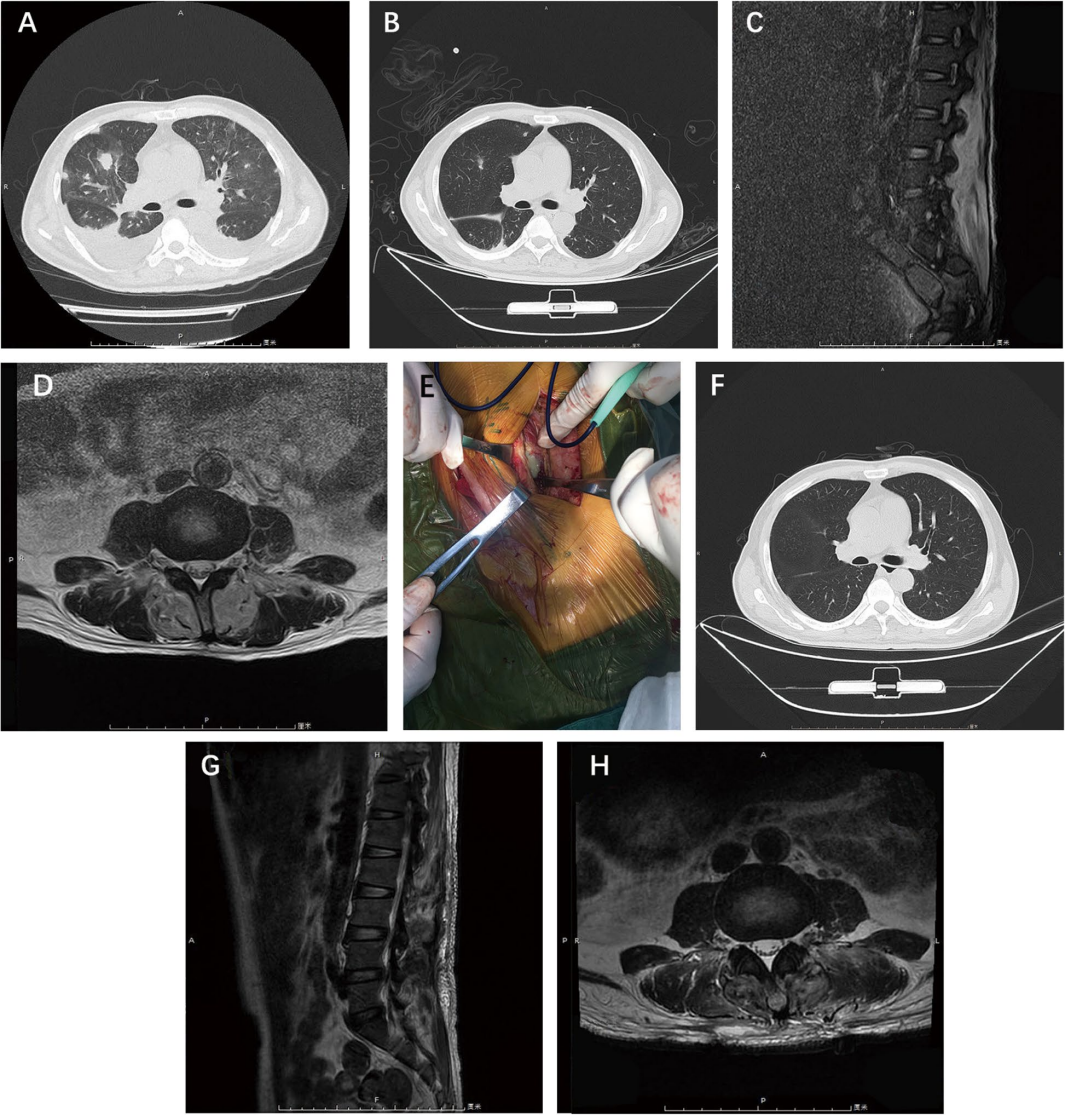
A, B and C, D: chest CT and MRI of the lumbar spine before surgery. F and G, H: after surgery. E: Operative field
